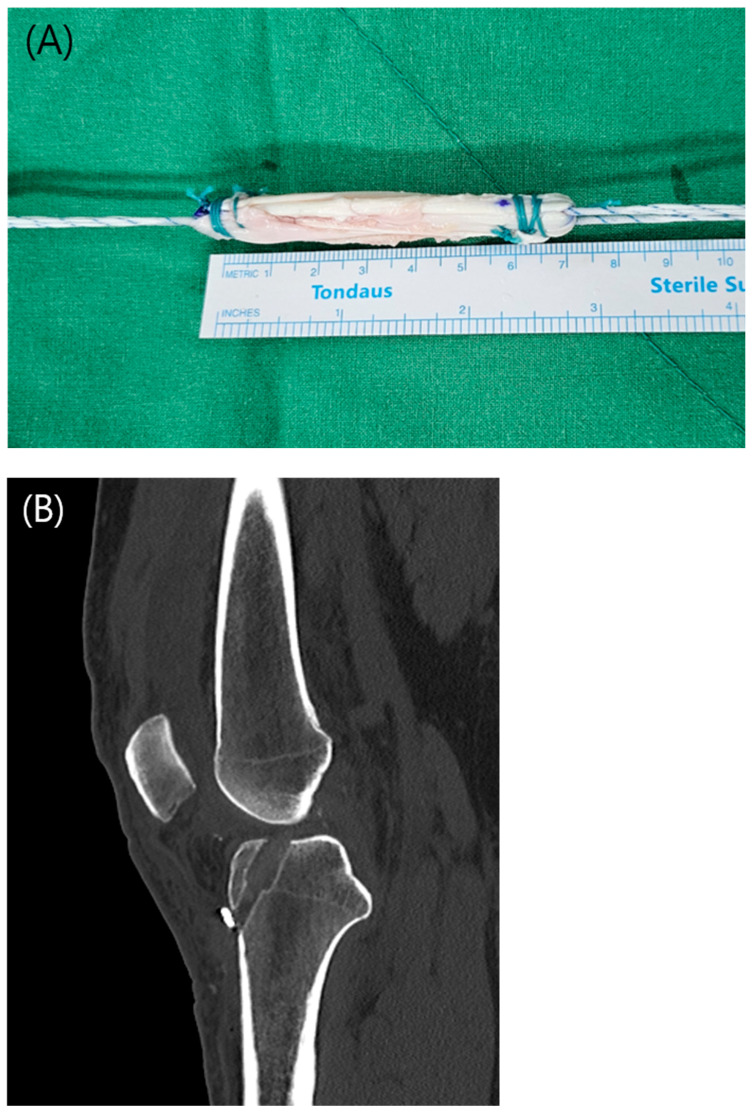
(A) Final construct of a 6-strand hamstring autograft. (B) Sagittal computed tomography image showing the configuration of the tibial tunnel after all-inside ACL reconstruction.
